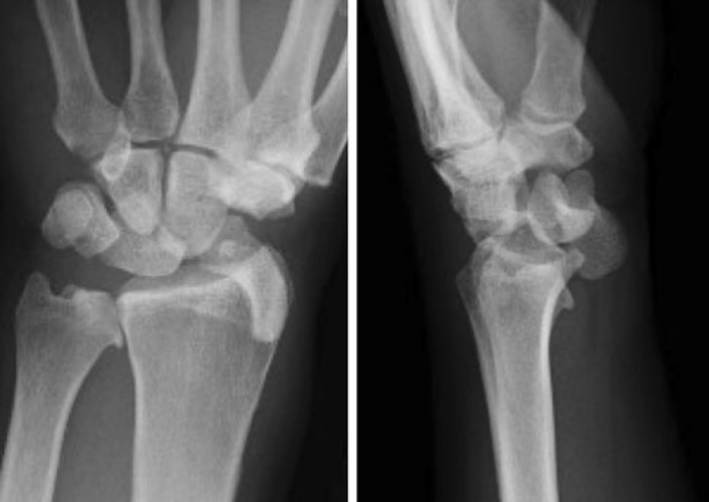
Radiographs at initial diagnosis showing palmar-divergent dislocation of the scaphoid and lunate
